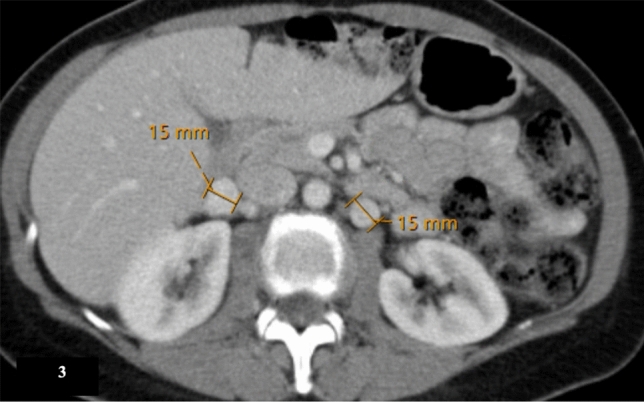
Dilated gonadal veins in patient with pelvic venous disorder. 51-year-old multiparous female presenting with more than 7 months of constant, dull pelvic pain. She later underwent embolization of the left gonadal vein with resolution of chronic pelvic pain symptoms. Axial contrast-enhanced computed tomography with measurement calipers (orange) shows a right gonadal vein measuring 15 mm and left ovarian vein measuring 15 mm
